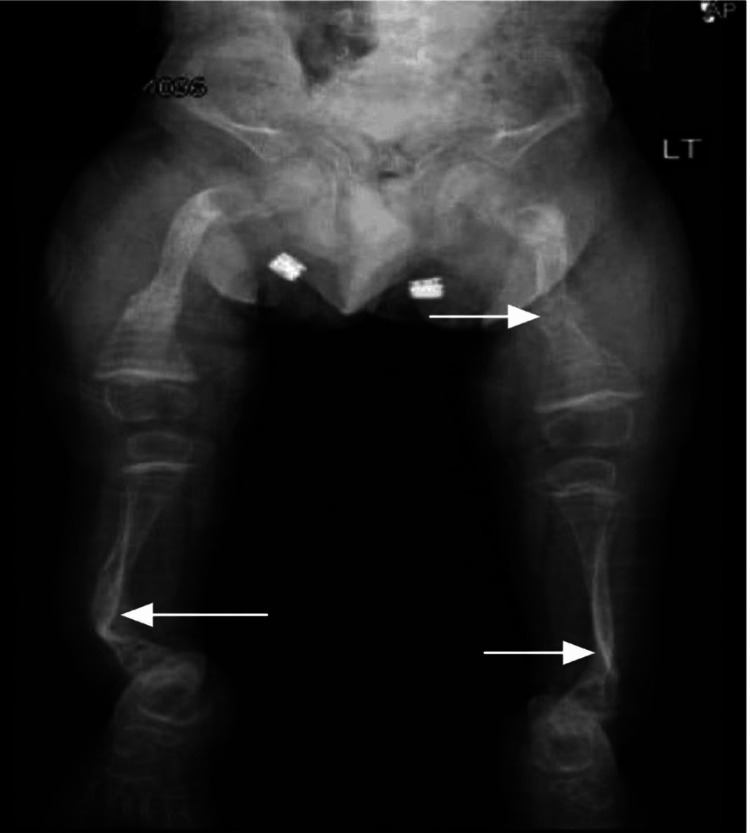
Plain radiograph (anteroposterior view) showing short and wide bones, thin cortices, rarefaction of trabecular bone, and osteopenia. Various bone deformities, including anterior bowing of the tibia (saber shin deformity), are observed. Arrows indicate multiple fractures in different stages of healing.
